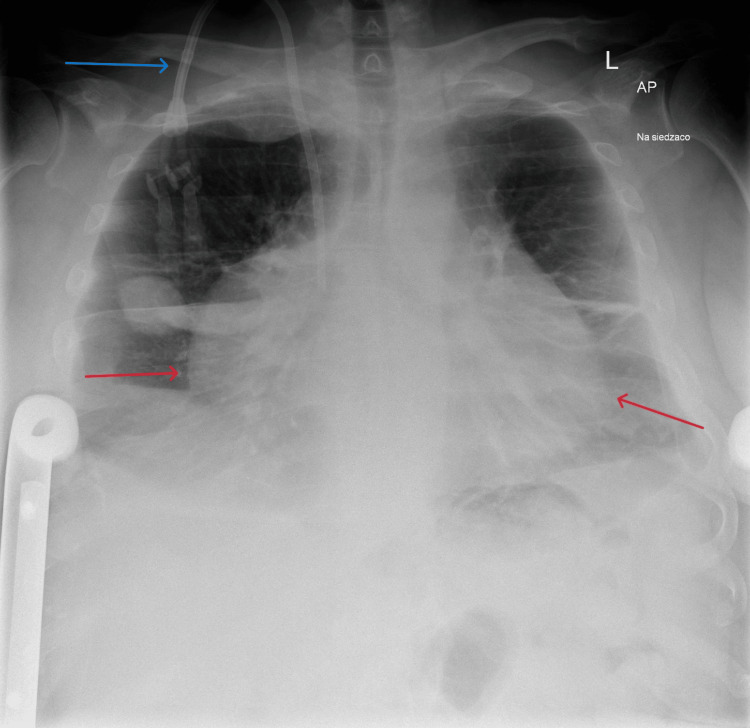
Chest radiograph obtained on admission. Demonstrated marked enlargement of the cardiac silhouette with a globular “water bottle” configuration consistent with massive pericardial effusion (red arrows). Additional findings included pulmonary vascular congestion and bilateral pleural effusions in the setting of profound hypervolemia secondary to interrupted hemodialysis. A tunneled permanent dialysis catheter is visible on the right side, inserted via the right internal jugular vein (blue arrow).
